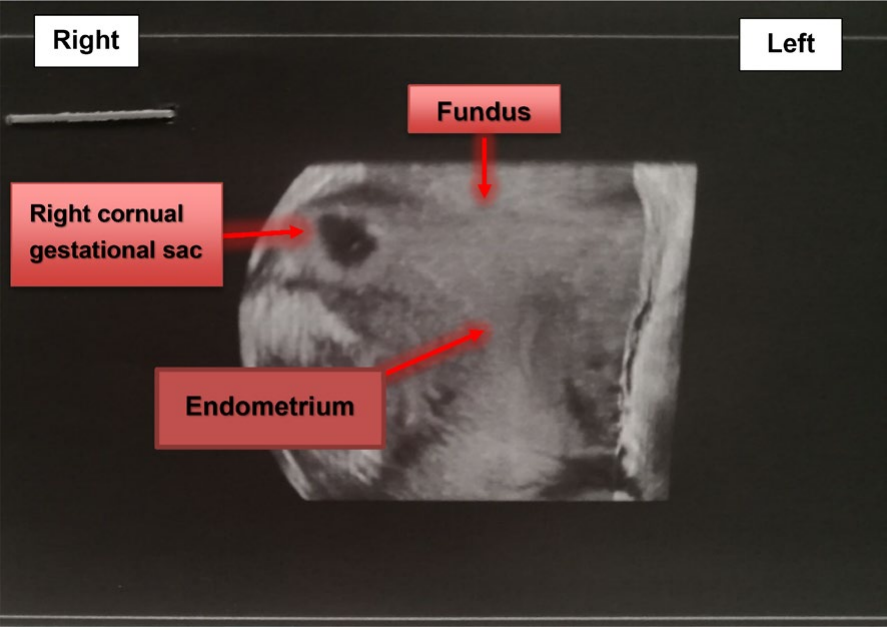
Three‐dimensional transvaginal ultrasound revealed that the gestational sac was not connected with endometrium.
